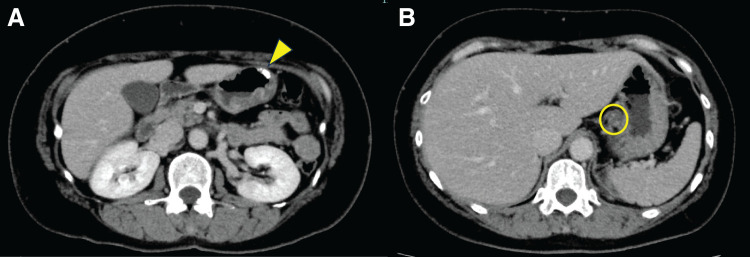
Preoperative CT imaging. (A) Wall thickening and partial calcification (arrowhead) are observed in the gastric body. (B) Enlargement of the lesser curvature lymph node suggesting lymph node metastasis (circle).
